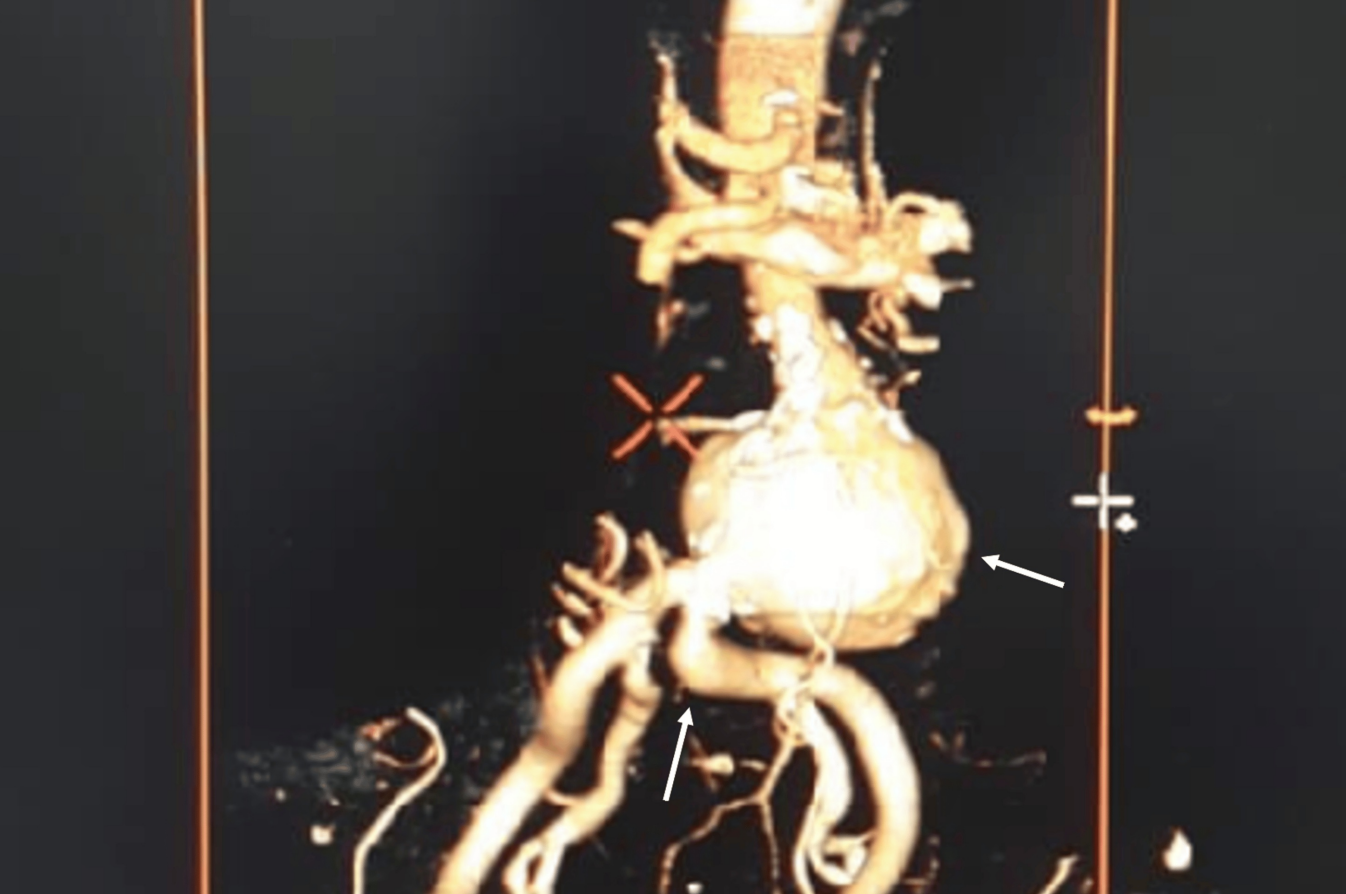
CT aortoiliac angiography revealed an infrarenal abdominal aortic aneurysm with severe tortuosity of the left common iliac artery.
